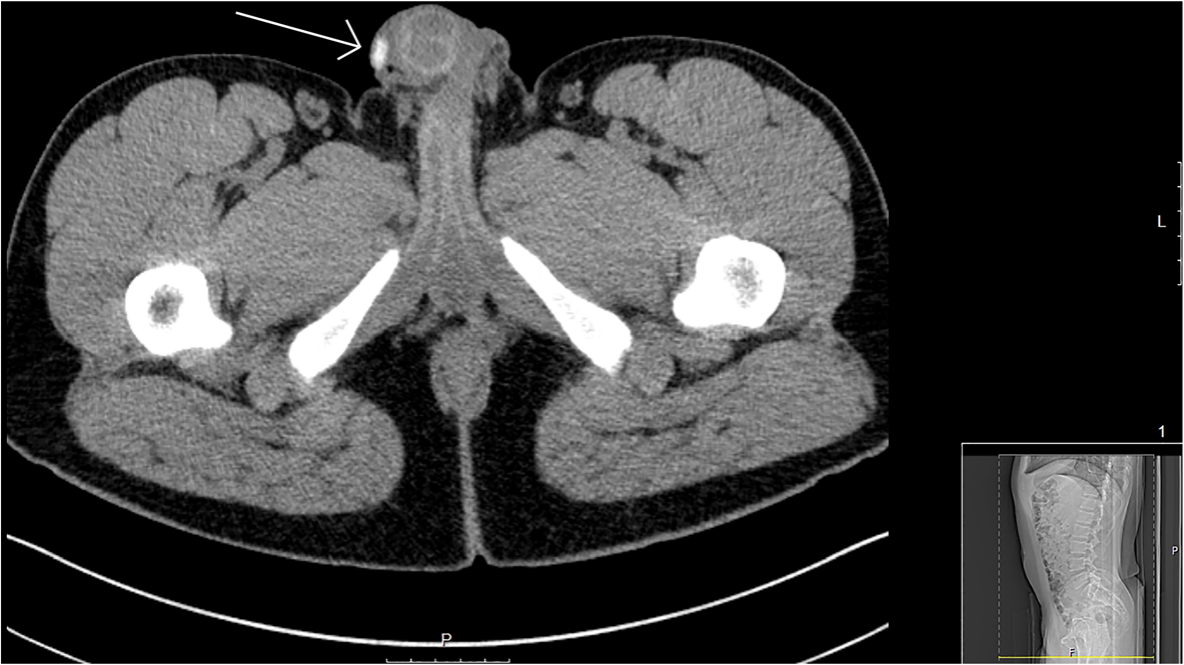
A computed tomography (CT) scan with radiopaque subcutaneous penile foreign body (white arrow) is present on this cut
